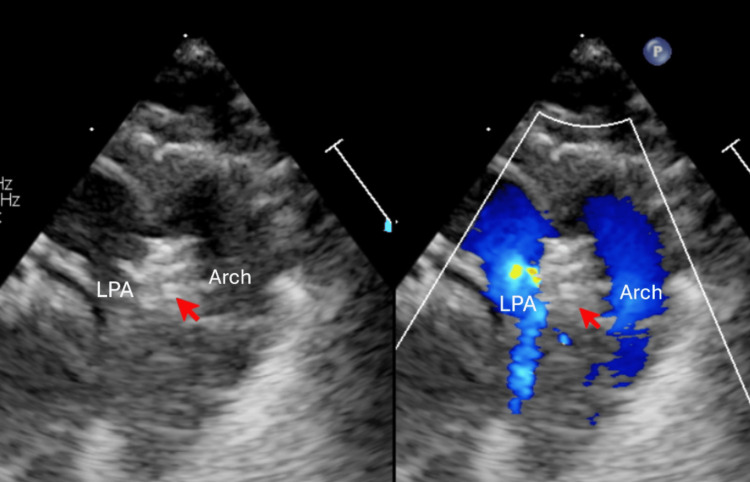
Echocardiography showed device position (indicated by red arrow) with color doppler no gradient in LPA and across the arch.
